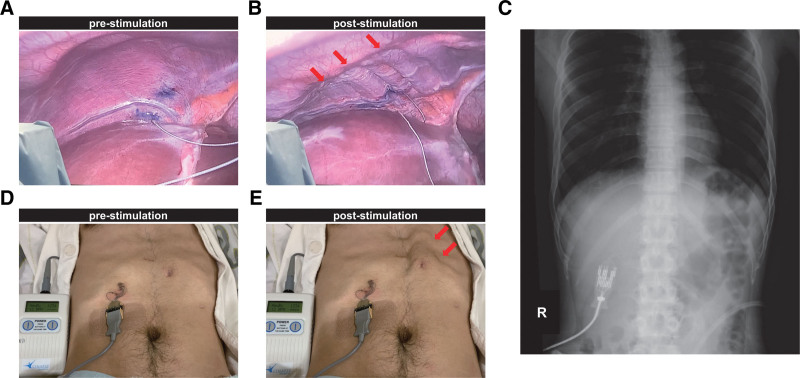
Laparoscopic images before (A) and after (B) diaphragm stimulation. An electrode was placed in the diaphragm at the point where maximal contraction of the diaphragm was identified after the diaphragm was mapped. Arrow heads show the contraction of the diaphragm poststimulation (B). (C) Chest and abdomen radiograph showing phrenic nerve stimulator electrodes connected bilaterally to a pulse generator. Postoperative appearance of the patient before (D) and after (E) diaphragm stimulation. The four implanted electrodes were tunneled to the external pulse generator that stimulated his diaphragm to allow ventilation. In addition to the diaphragm, muscle contraction of the rectus abdominis (arrow heads) can be seen on the exterior poststimulation.
